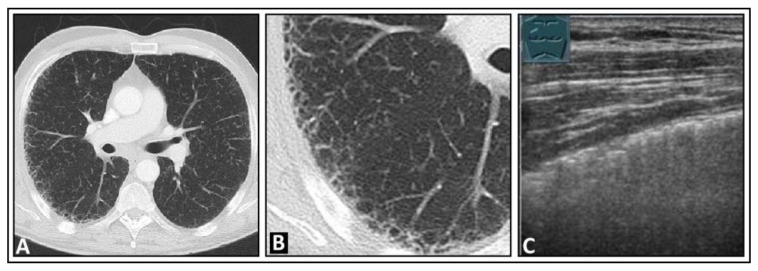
A patient with exertional dyspnea and confirmed idiopathic pulmonary fibrosis. (A) The chest computed tomography displays subpleural fine reticular changes in the upper lobe leading to honeycombing, with the right side (B) more affected than the left. (C) On lung ultrasound, multiple confluent B-lines are seen on the right side, with a rough lung surface.
